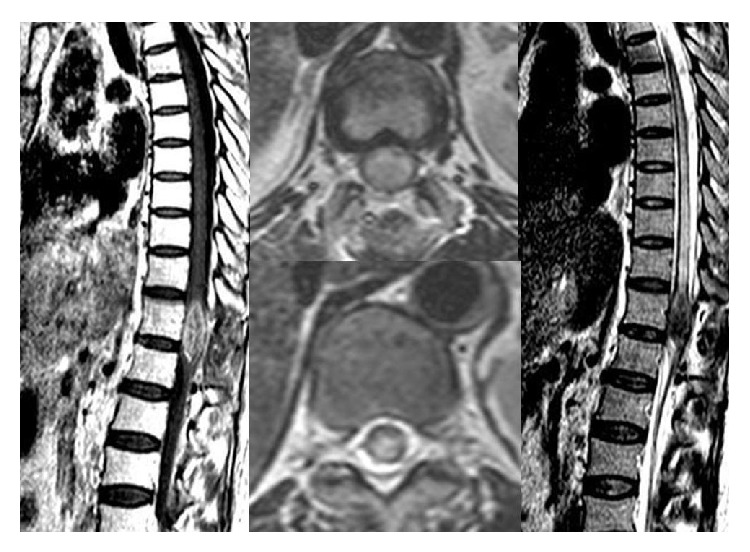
Spinal MRI showed an intramedullary and highly gadolinium enhancing lesion with regular borders.
